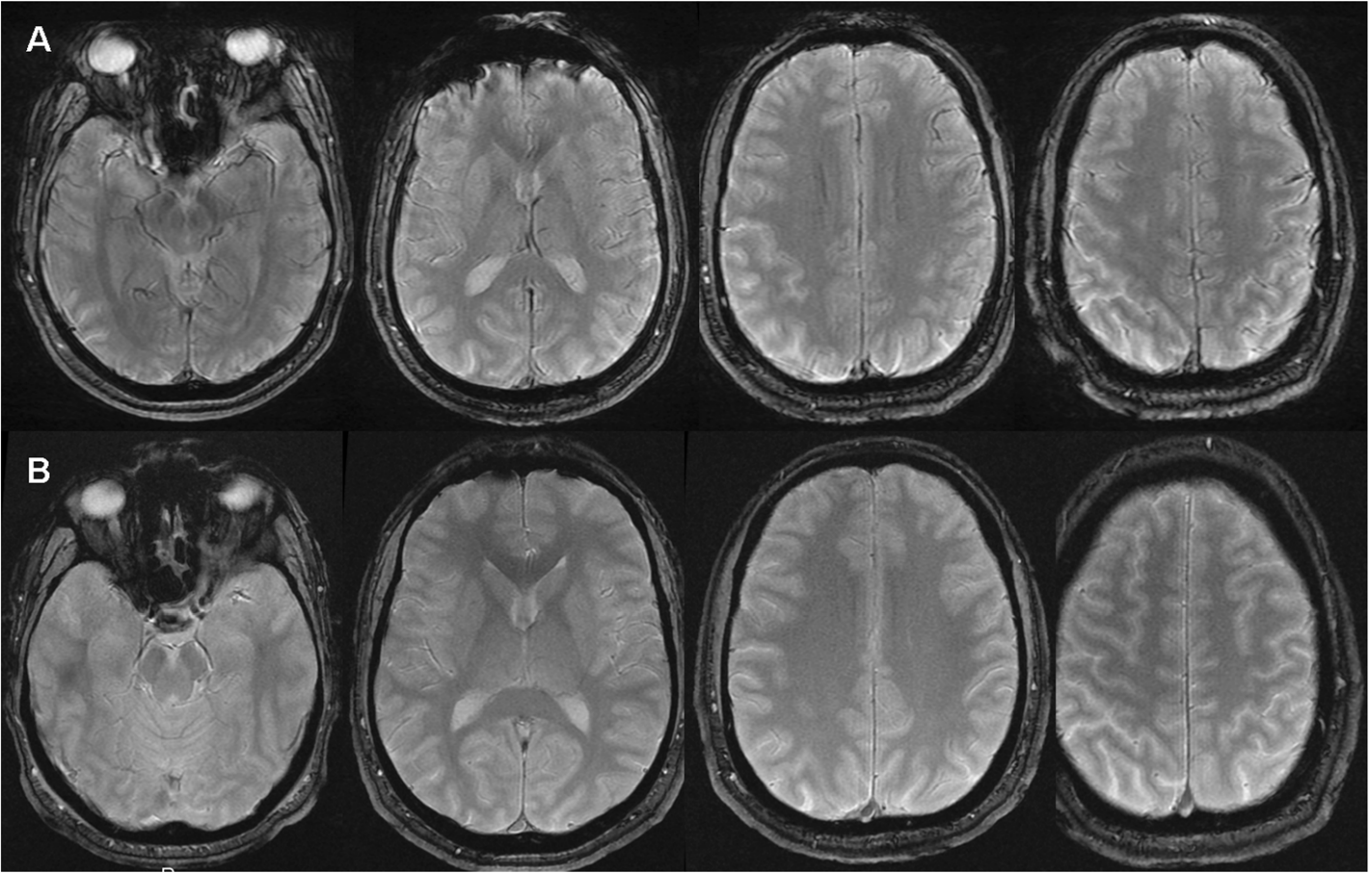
A gradient-echo T2*-weighted magnetic resonance image showing hypointense signals in both hemispheres of the brain but especially in the left hemisphere (A) and a normal gradient-echo T2*-weighted magnetic resonance image acquired five days after the migraine attack (B).
